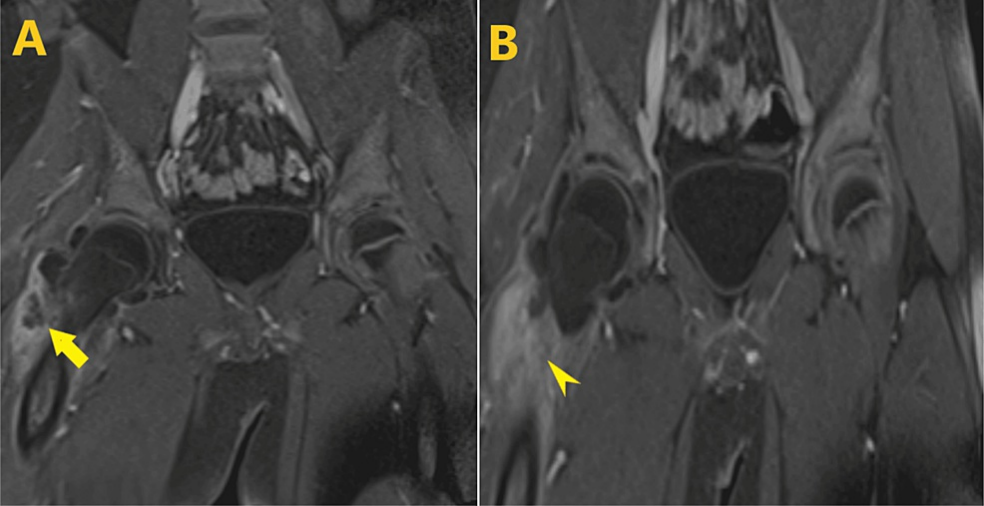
(A) T1-weighted enhanced MR images in the coronal plane showing thin enhancement of the synovium (arrow); (B) associated with surrounding soft tissue edema and enhancement (arrowhead)
